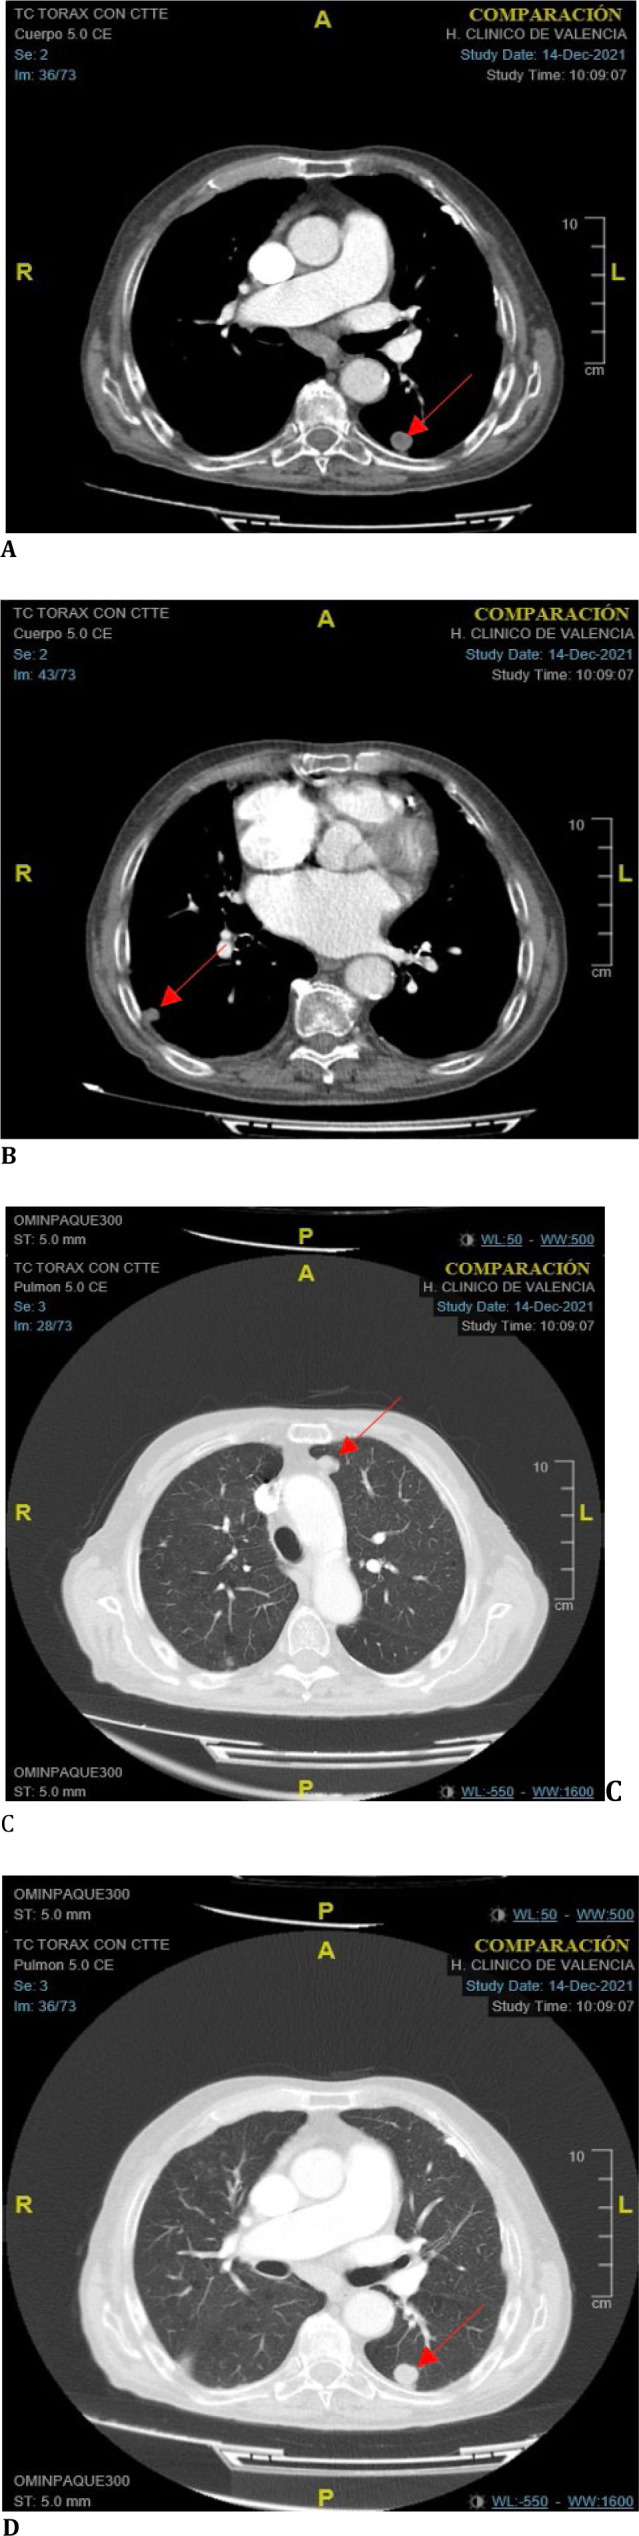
A-D Chest CT revealing multiple bilateral pulmonary nodules and mediastinal lymphadenopathy suggestive of metastatic disease
